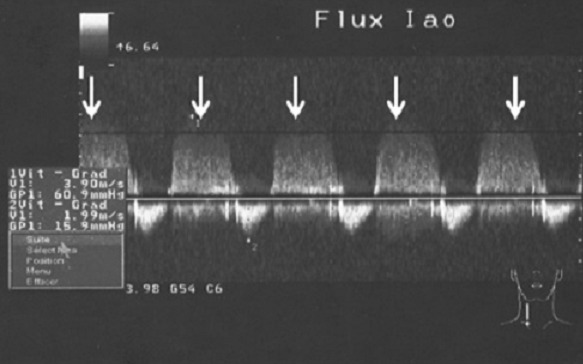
Echographie doppler objectivant un flux d’insuffisance aortique (flèches)
